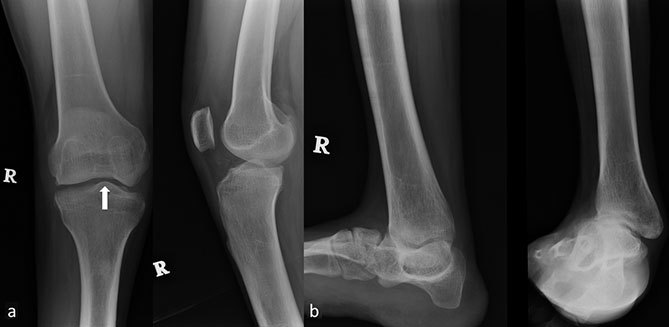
(a) Frontal and lateral radiographs of the right knee show a convex tibial eminence and a hypoplastic tibial spine (arrow). The lateral femoral condyle appears hypoplastic with anterior translation of the right tibia on lateral radiograph. (b) Frontal and lateral radiographs of the right ankle show severe ankle joint deformity.
